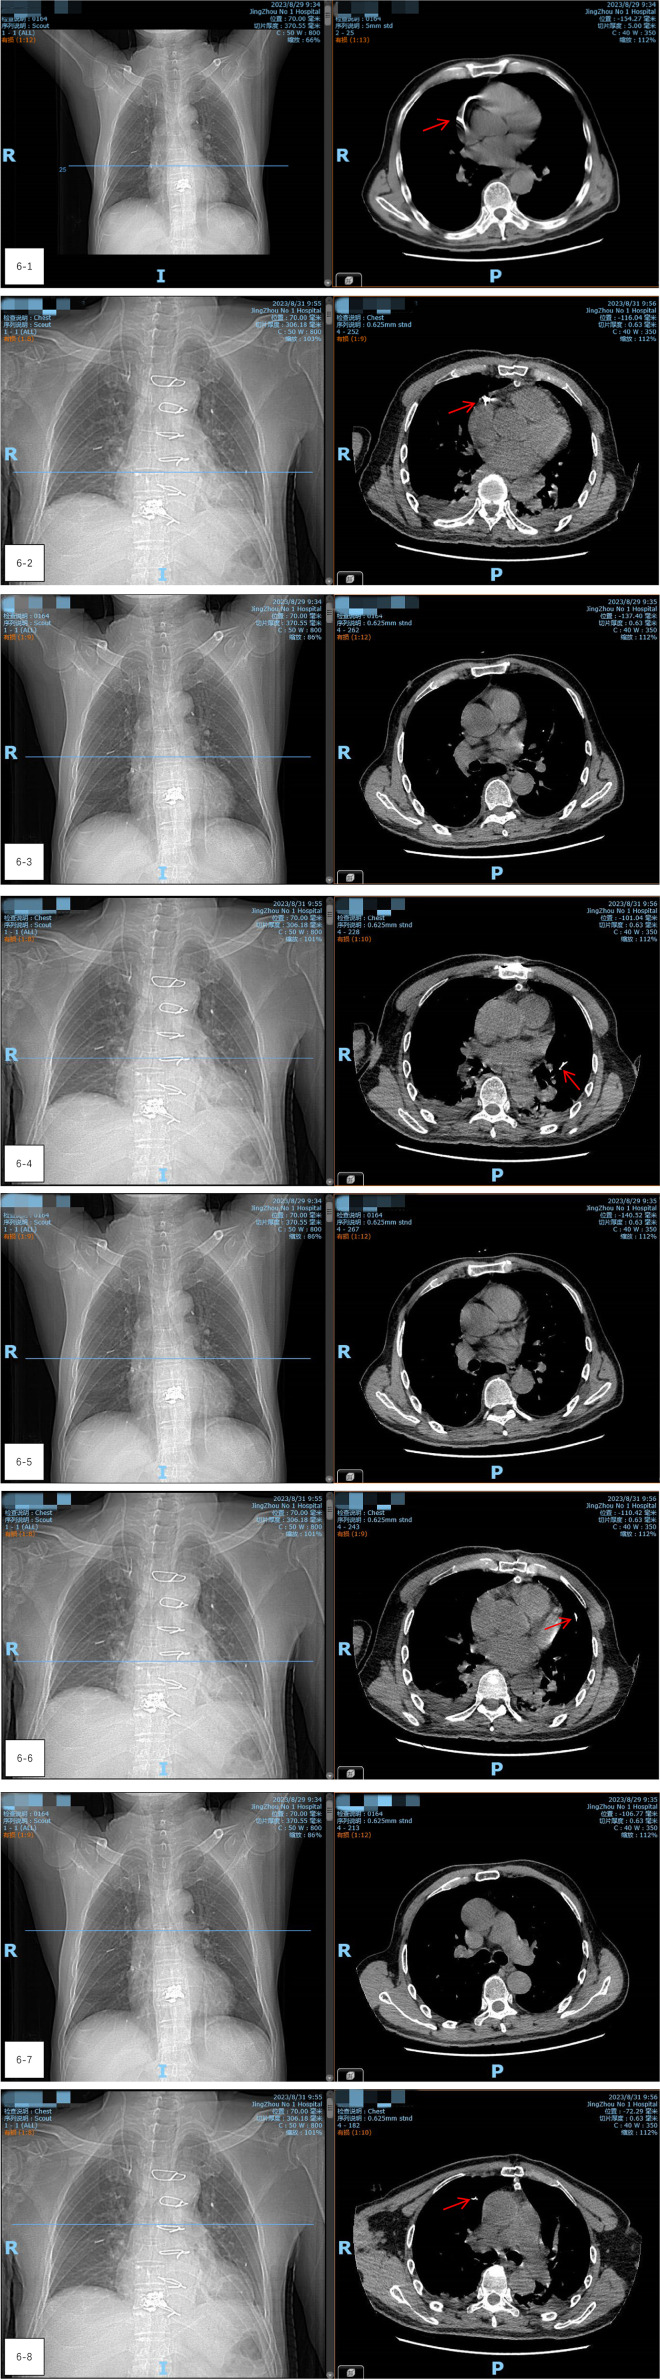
(6-1–6-8) A follow-up chest CT scan on the second day after thoracotomy, compared with the preoperative CT, confirmed a significant reduction in the high-density shadow in the heart and the appearance of new shadows in the lung, verifying the migration of fractured cement into the pulmonary artery.
